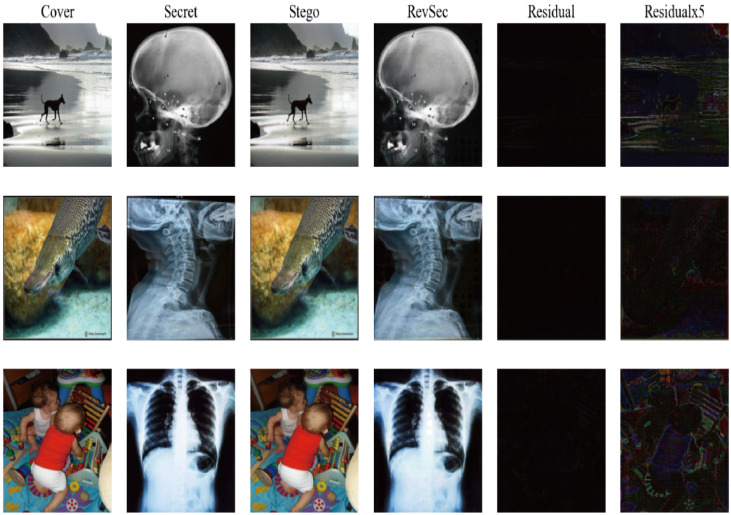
The test results of our proposed scheme on CT images.
